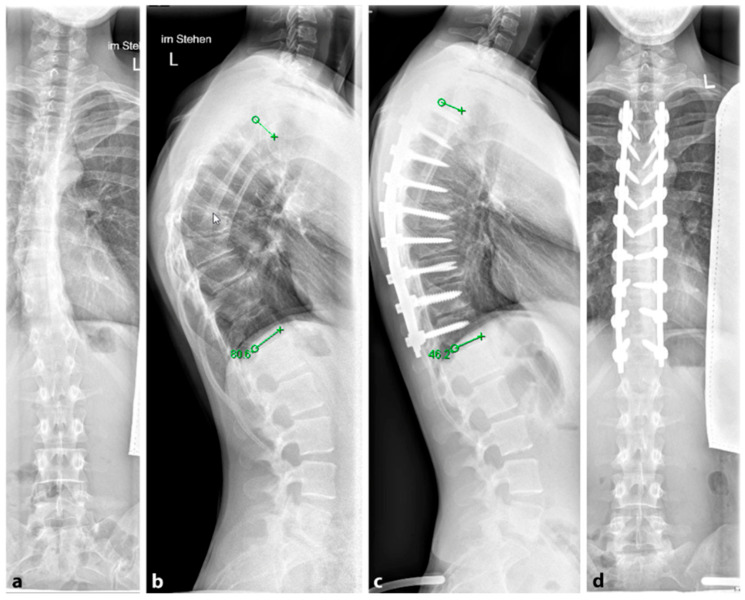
(a,b). Preoperative and (c,d). postoperative radiographs after corrective spondylodesis from T4 to T12 with ventral release, correcting the Stagnara angle from 80 to 46 degrees (reprinted with permission [23]).
